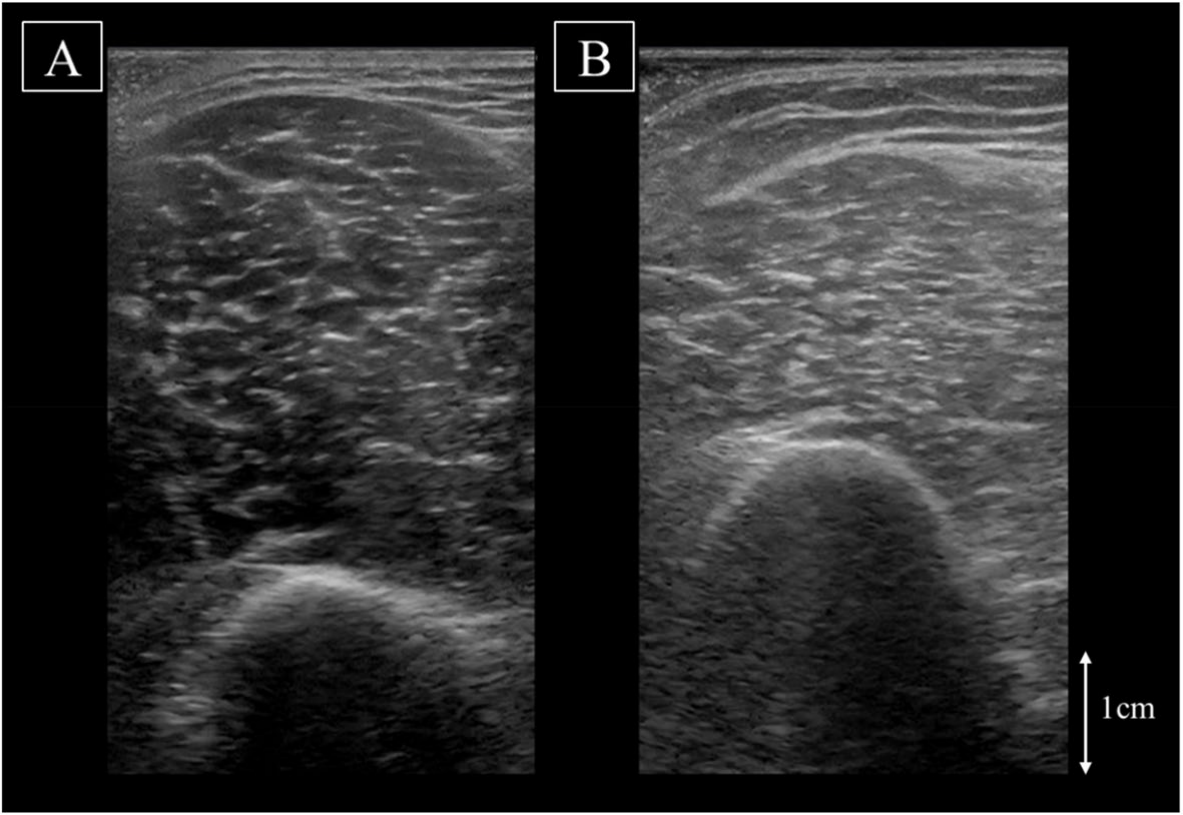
Representative ultrasound images measured in the sitting posture of A young and B older individuals. The young participant exhibits thick MT and low EI, while the older participant exhibits thin MT and high EI. The higher density of white values in the older participant reflects increased intraMAT
